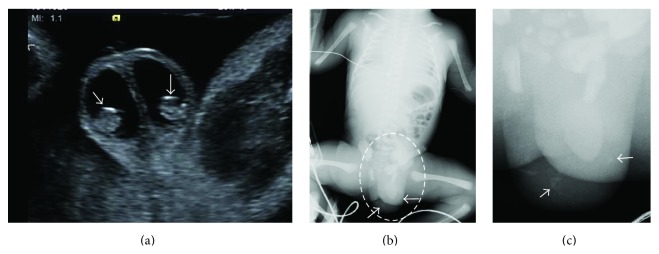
Prenatal ultrasonographic and postnatal radiographic findings. Prenatal ultrasonography revealed bilateral hydrocele with peritesticular calcification at 28 weeks' gestation (a), while postnatal radiography demonstrated scrotal calcification without abdominal calcification (b, c). (c) was an enlarged view of the dotted circle in (b). Arrow indicated calcification.
